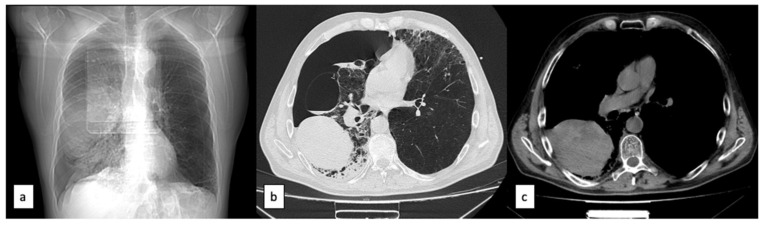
Scans indicate a right basal pneumothorax with opacification of bullous lesions: (a) X ray; (b) CT-lung window; (c) CT-chest window.
